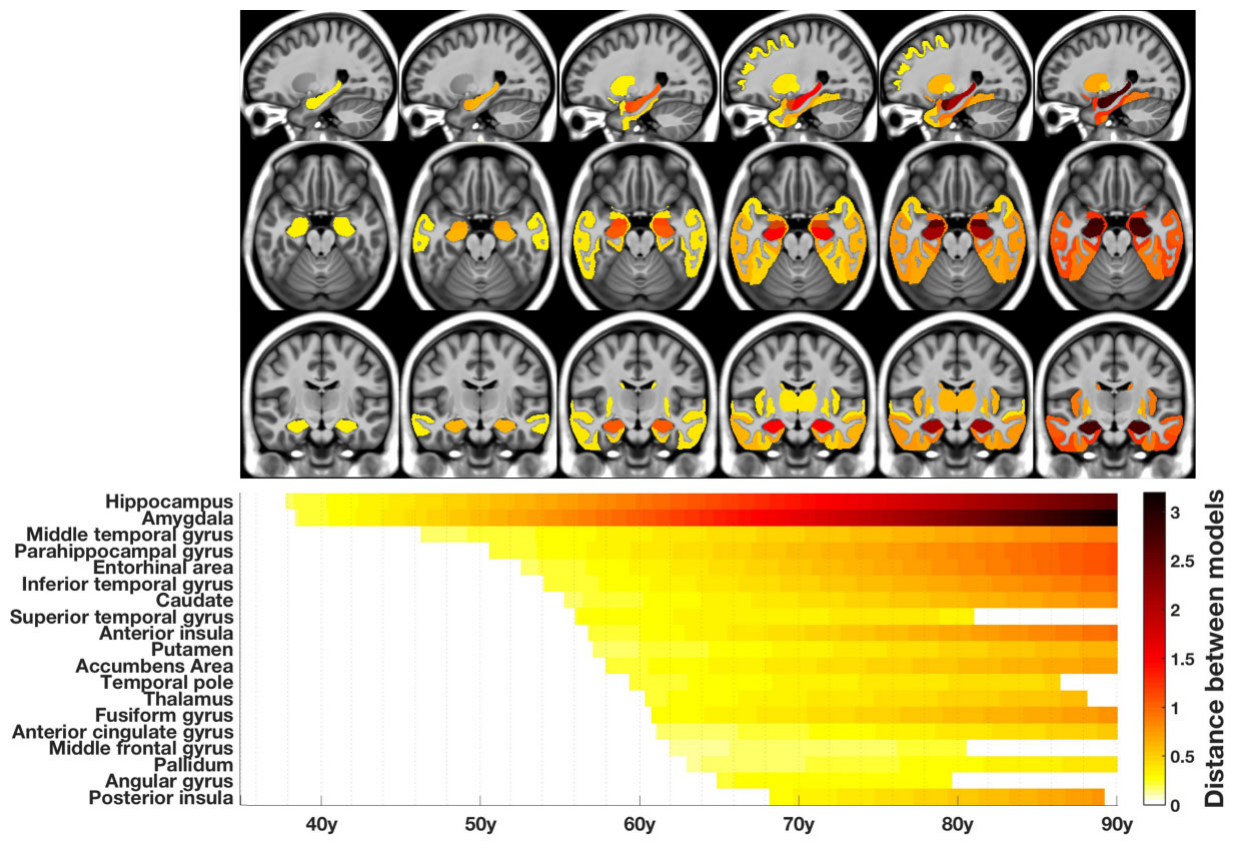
The MRI staging scheme of structural progression of Alzheimer’s disease. The figure represents the chronological progression of distances between healthy and Alzheimer’s disease trajectories. The severity of structural divergence is colour-coded according to the bar at the bottom right of the figure.
